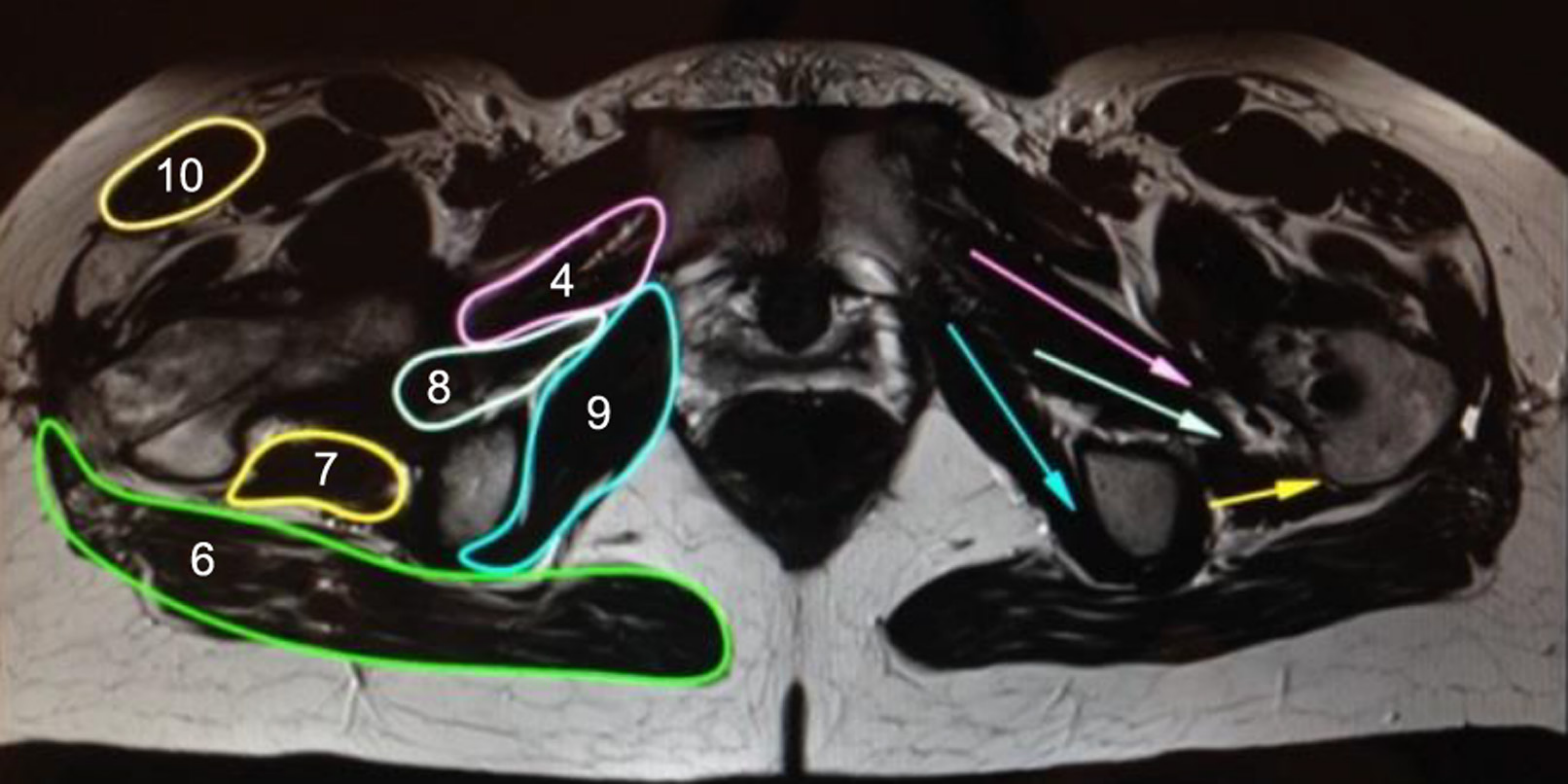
Axial T1‐MRI scan with outlines (patient right) and straight line markups (patient left). 4 Triceps coxae; 6 gluteus maximus; 7 quadratus femoris; 8 piriformis; 9 obturator internus before deflection; 10 tensor fasciae latae.
